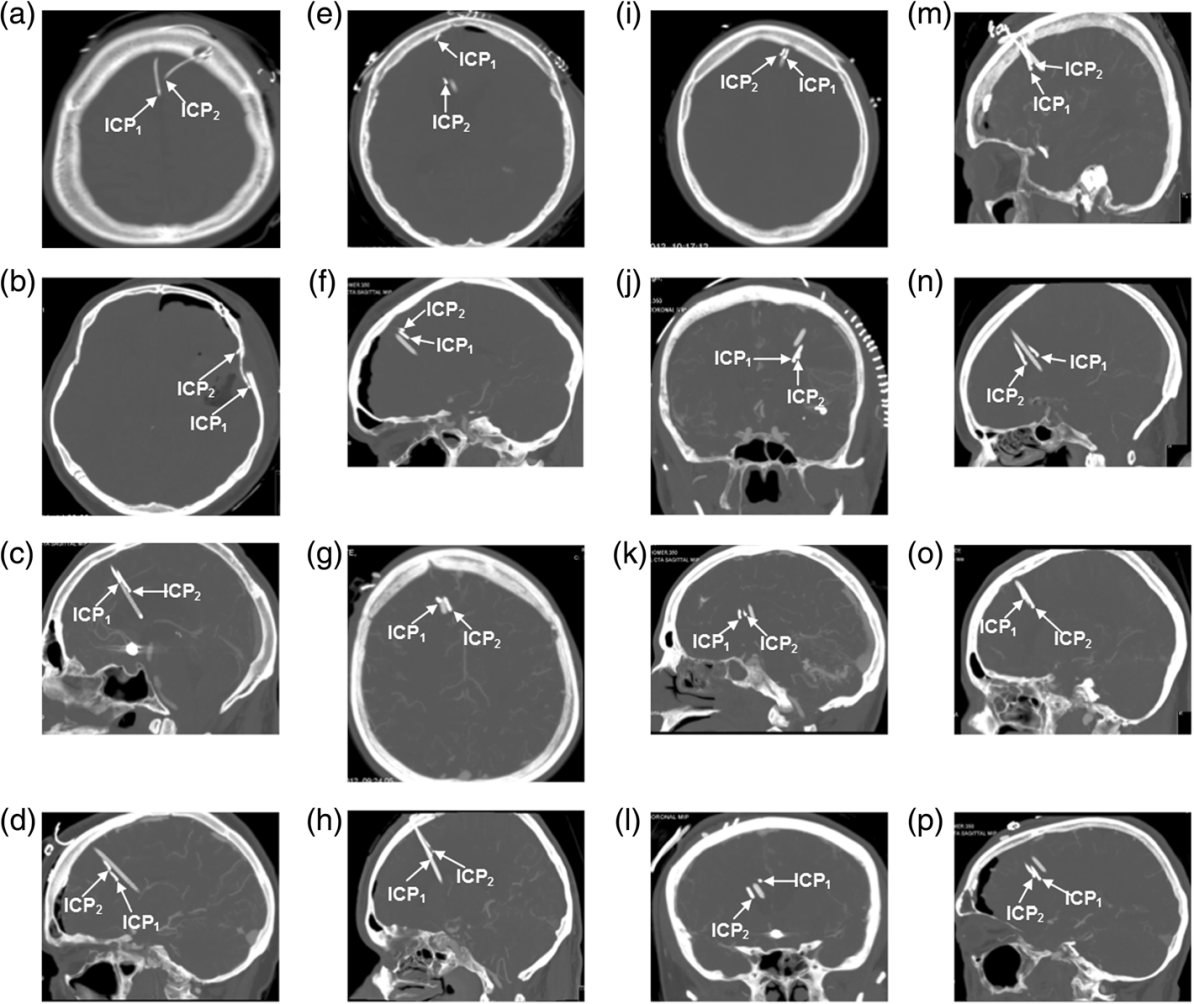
Locations of ICP sensors 1 and 2 on cerebral computer tomography (CT). The CT scans showing the two ICP sensors (ICP_1_/ICP_2_) in (a) Patient 1, (b) Patient 2, (c) Patient 3, (d) Patient 4, (e) Patient 5, (f) Patient 6, (g) Patient 7, (h) Patient 8, (i) Patient 9, (j) Patient 10, (k) Patient 11, (l) Patient 12, (m) Patient 13, (n) Patient 14, (o) Patient 15, and (p) Patient 16.
